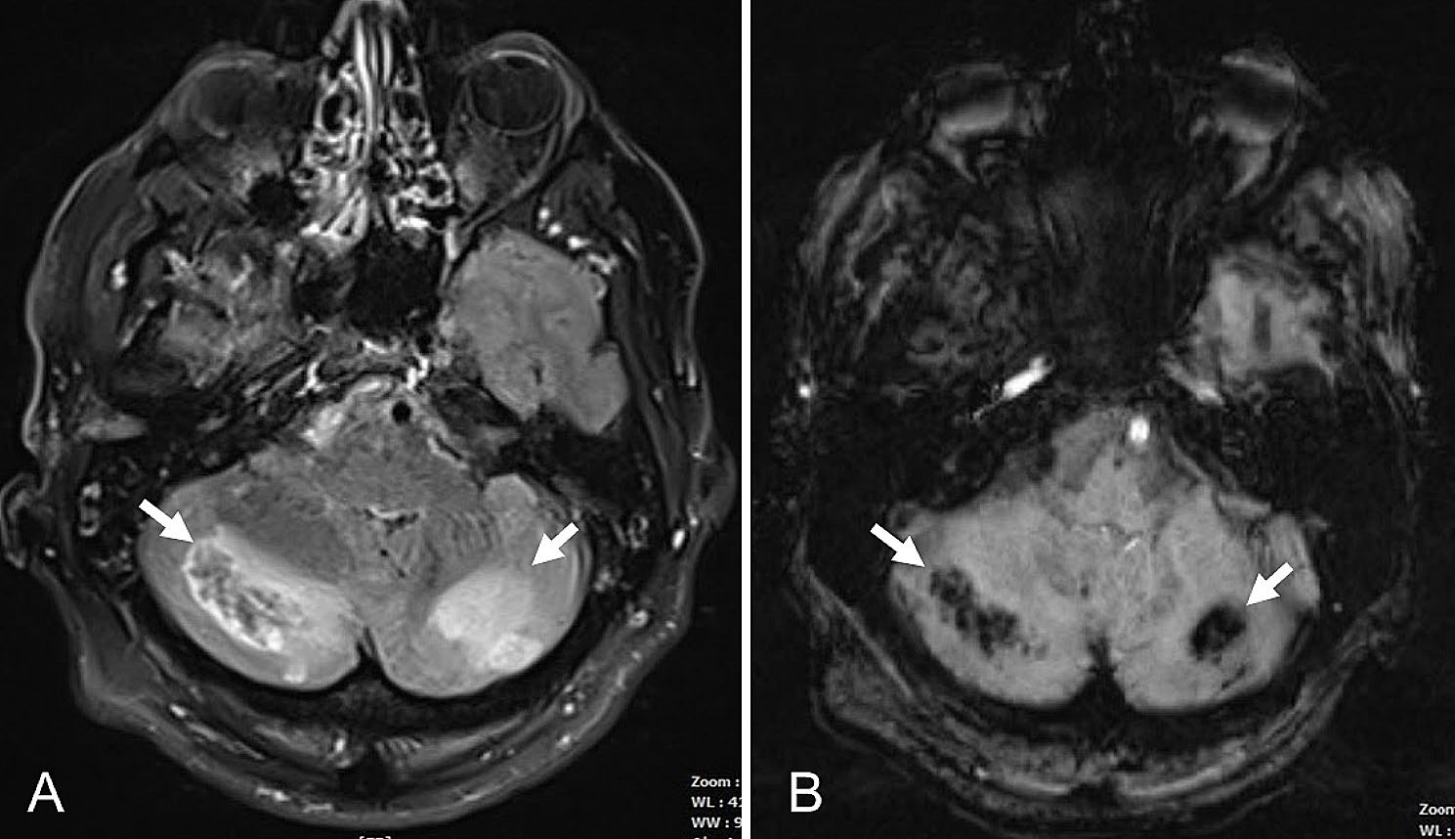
A-B: MRI scan on day 6. FLAIR (A) and susceptibility weighted (B) images, showing bilateral hemorrhagic (B) cerebellar lesions, complementing the typical imaging findings of CHANTER syndrome in Fig. 1
